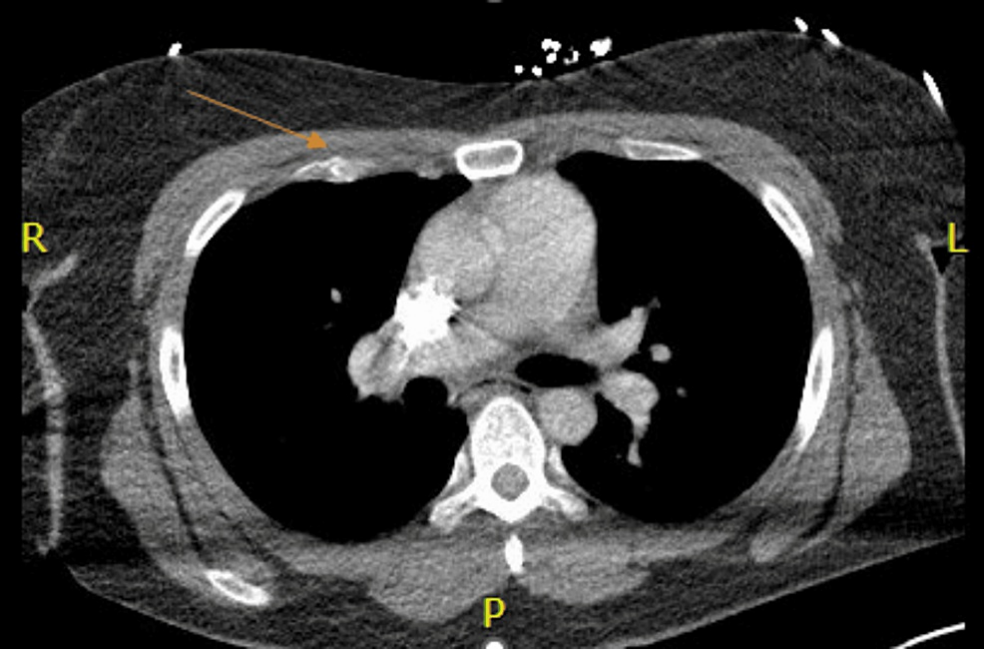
CT axial scan of the chest with intravenous contrast - image 1 The orange arrow shows nondisplaced anterior rib fractures CT: computed tomography
